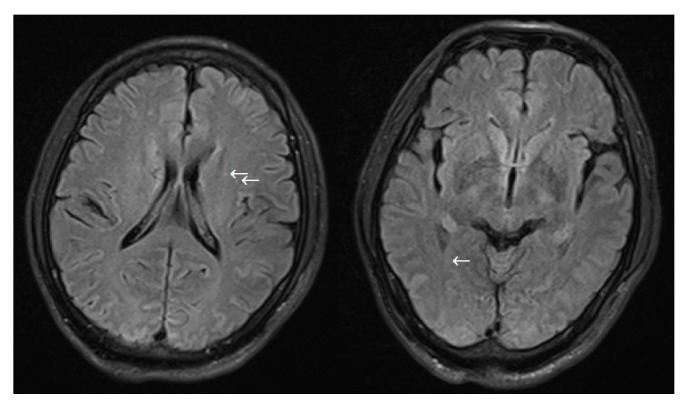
Diffuse hyperdense signals (*←*) on the cerebral MRI, indicative of acute cerebral infarction.
